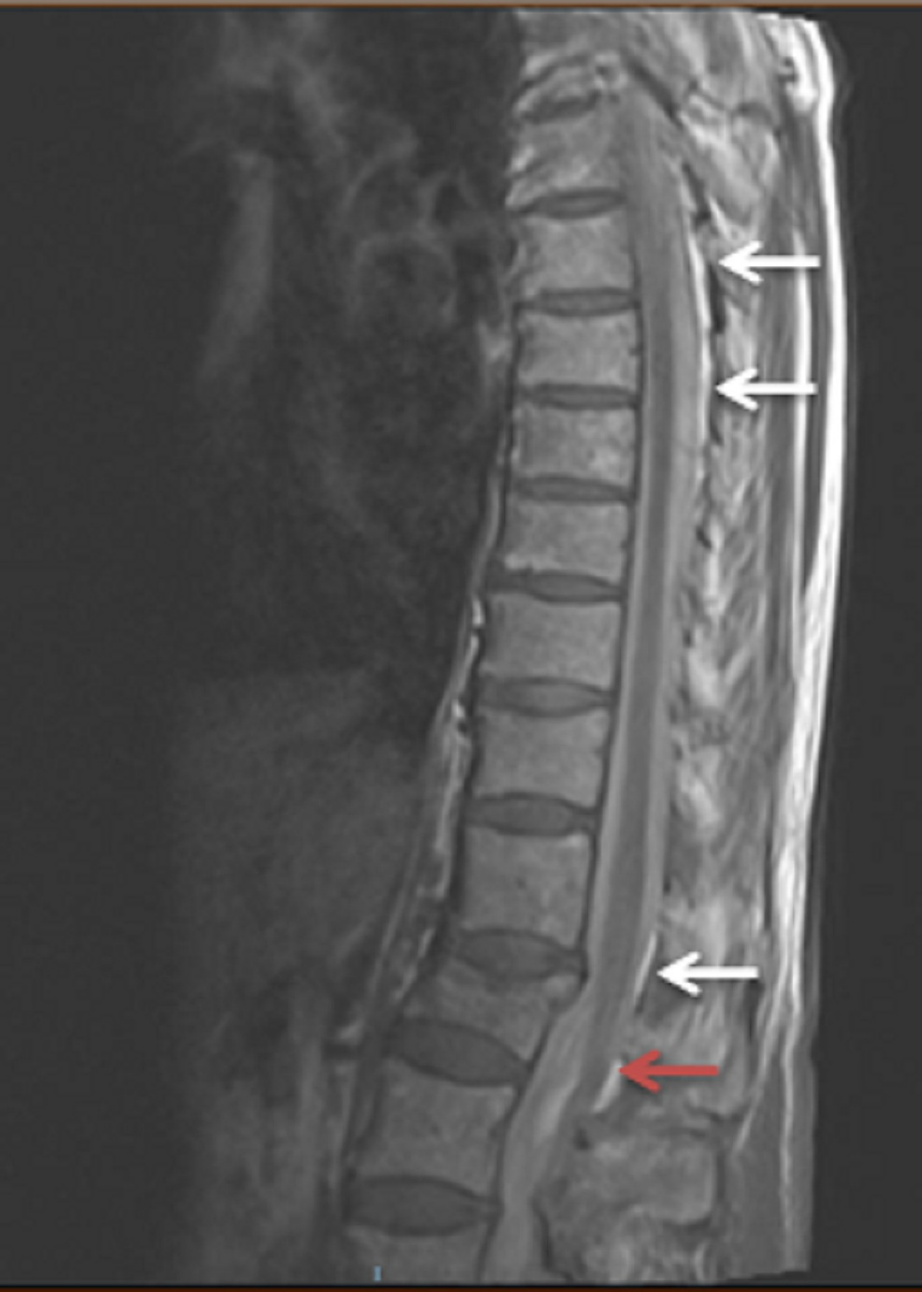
Sagittal T1-weighted sequence lumbar spine. The spinal subarachnoid haemorrhage is demonstrated as abnormal high signal intensity posterior to the cord extending distally within the thoracic (white arrows) and lumbar spine (red arrow)
